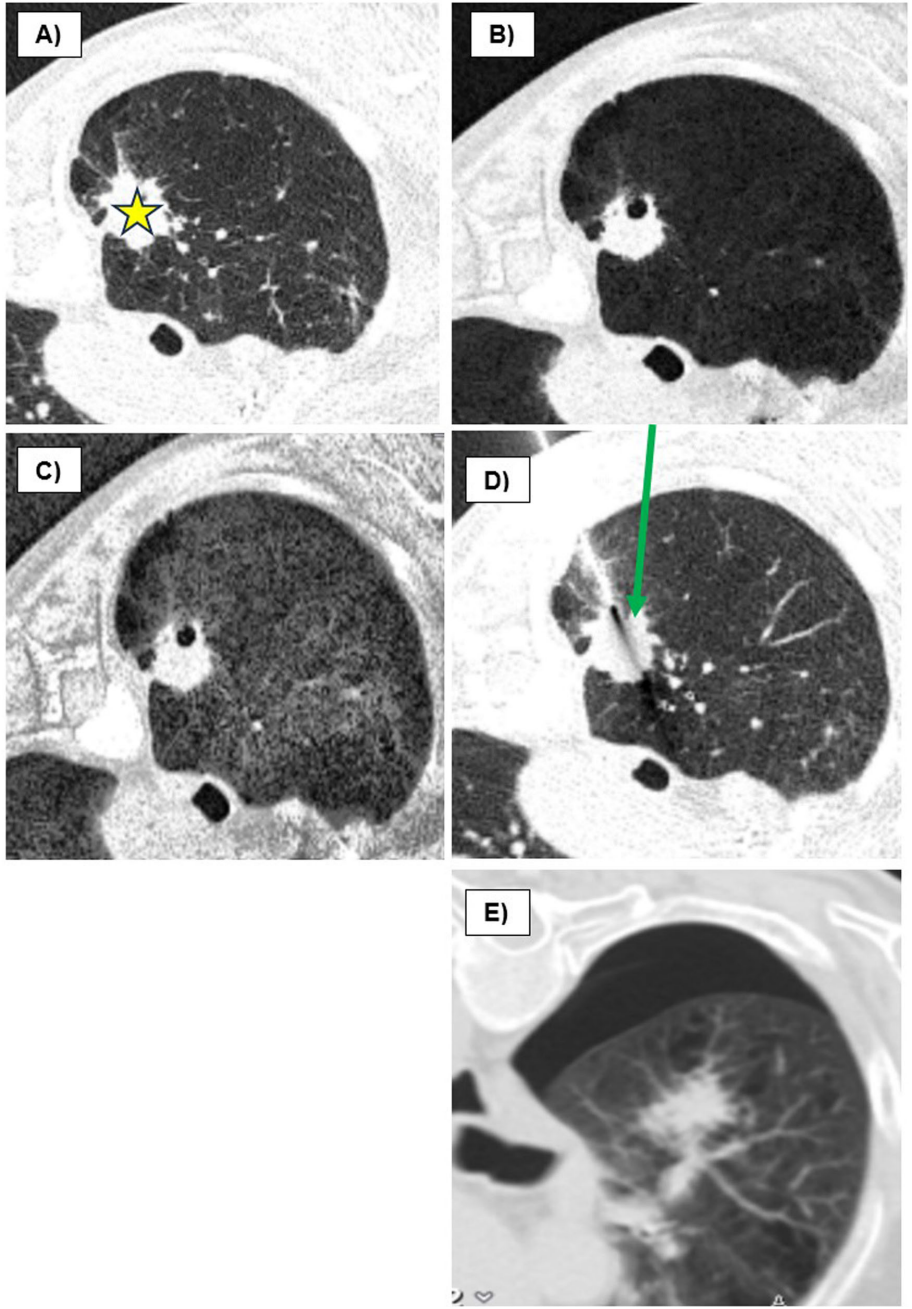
Evaluation of access routes using MinIP imaging in CT-guided lung biopsies. A Native scan is shown in the lung window (LW) before the biopsy. Malignant mass in the right upper lobe (asterisk). The patient is in a semi-prone position. B MinIP shows already inhomogeneous lung parenchyma. C MinIP with contrast enhancement shows damaged lung parenchyma in the later access route. D Coaxial needle ending directly in front of the target lesion. In comparison, the potentially safer access route (green arrow). E Pneumothorax and pulmonary hemorrhage along the needle tract and perilesionally. Pneumothorax might have been prevented if the initial conditions had been considered prior to the procedure
